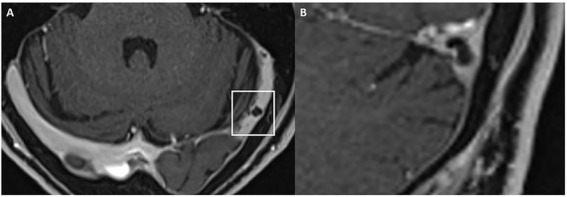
Left transverse sinus stenosis. Axial (A) and coronal (B) 3D T1w post-contrast MRI showing an arachnoid granulation in the left transverse sinus consistent with an intrinsic stenosis.
